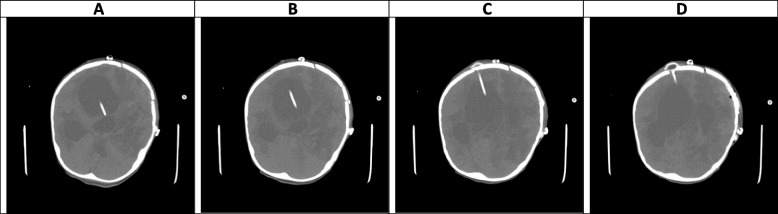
(the Brain CT SCAN without contrast, day 44). A-D All of the mentioned findings at the previous CT SCAN are present at this study, including significant hydrocephalus. It means either some degree of EVD malfunction or severity of hydrocephalus
